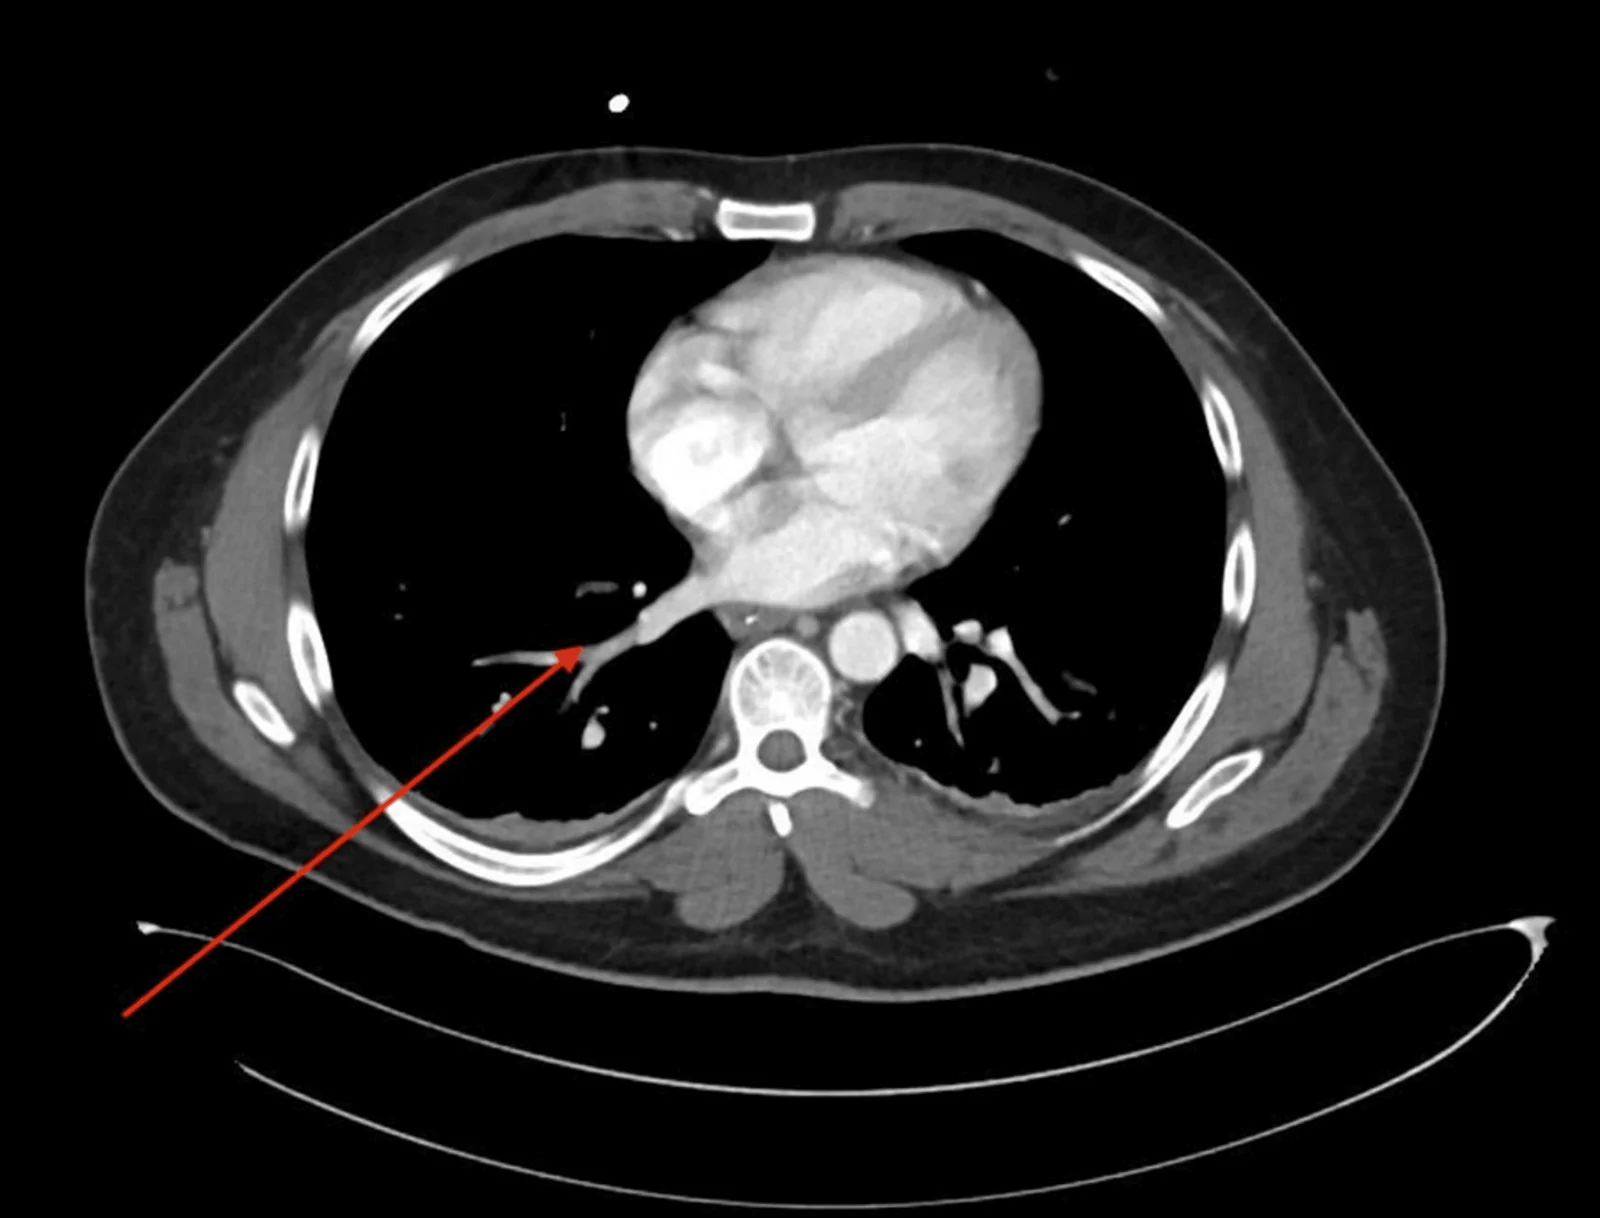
Contrast-enhanced computed tomography pulmonary angiogram (axial view) showing a filling defect in a right sub-segmental pulmonary artery branch, consistent with sub-segmental pulmonary embolism.
